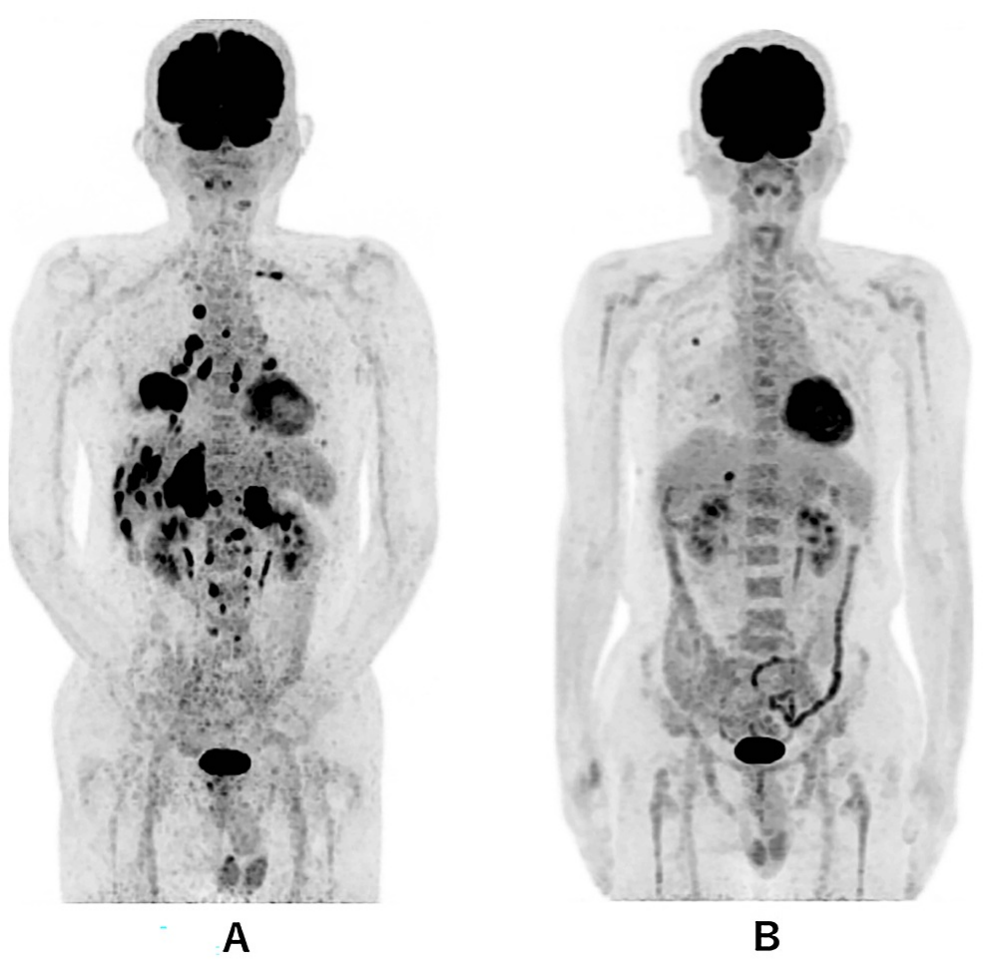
Whole-body PET-CT A: PET-CT at diagnosis showing multiple bilateral intrapulmonary metastases, multiple liver metastases, bilateral adrenal metastases, and multiple bone metastases, in addition to the primary tumor in the right lower lung. B: Whole-body PET-CT on day 479, showing two small metastases in the right lung and one in the liver.
